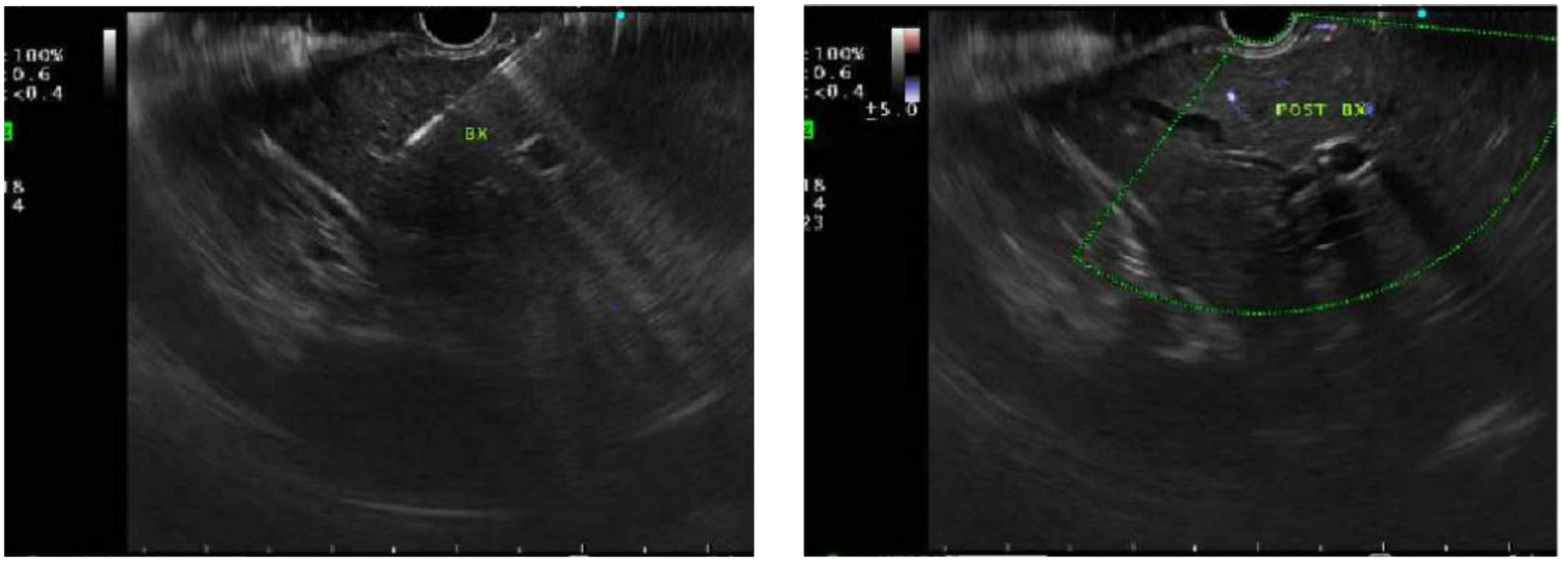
EUS-guided liver biopsy (left) and post-biopsy under Doppler imaging (right).
